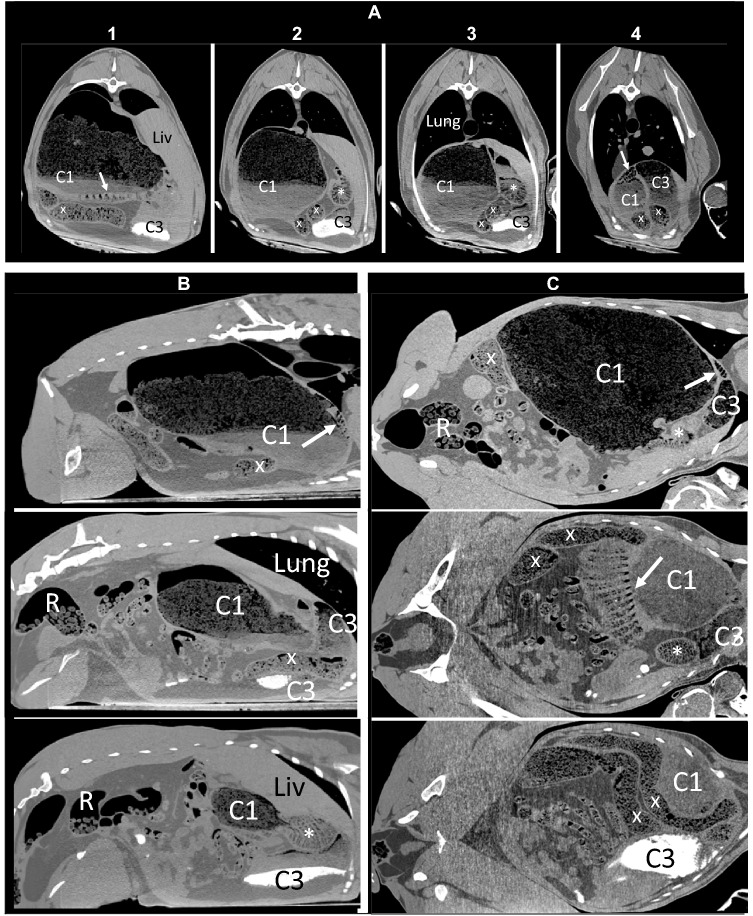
Computed tomography images of thorax and abdomen of a llama (*Lama glama*) fed a pelleted diet that included sand and grass hay for six weeks. Images represent A vertical slices in caudo-cranial view. The numbering corresponds to the dashed lines in Fig. 2, with the most cranial image on the right and the most caudal on the left; B sagittal slices in view from the right side, from the left (top) to the right part (bottom); C horizontal slices in dorso-ventral view, from the dorsal (top) to the bottom part (bottom). Note the dorso-ventral contents stratification in the C1 (with air, particulate matter with gas inclusions, and a fluid layer) and the glandular sacs (indicated by arrows). The C2 lies on the right side in the middle of the C1 (indicated by *) with its reticulated structure clearly visible. The C3 is cranial and ventral to the C2, and contains radiodense material in its ventral portion. Colon indicated by x, rectum filled with formed faeces by R. Liv = liver
